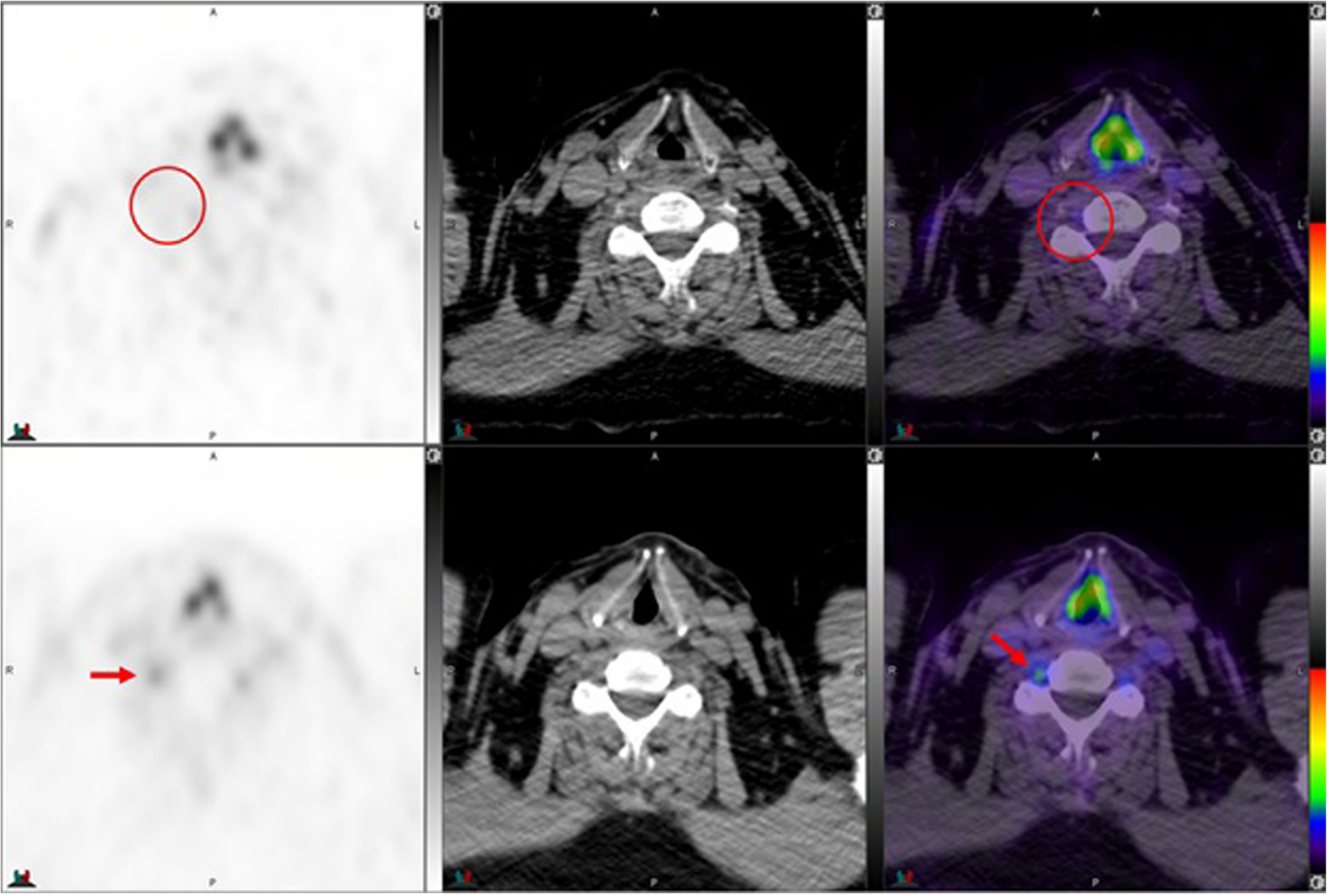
Top panel ^68^Ga-PSMA-11: axial PET, axial CT and axial fused PET/CT. Region of the Right lower cervical ganglion (circle). Lower panel ^18^F –DCFPyL: PET, CT and PET/CT. Right lower cervical ganglion uptake (SUVmax = 2.5) noted with ^18^F –DCFPyL (arrow)
